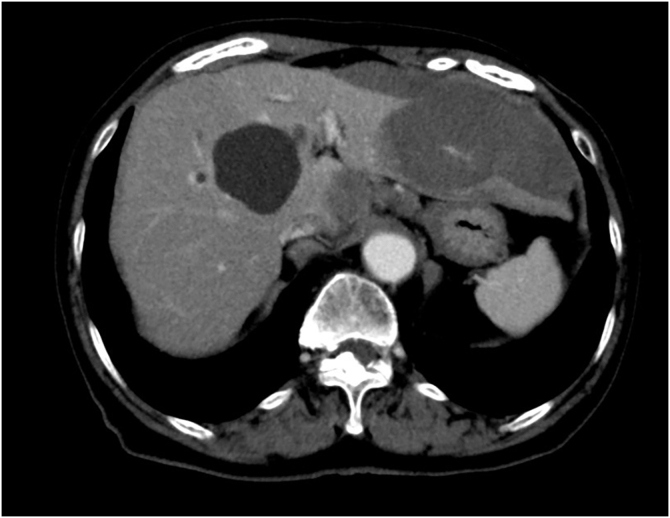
Abdominal CT showed a rupture of the liver metastases.
